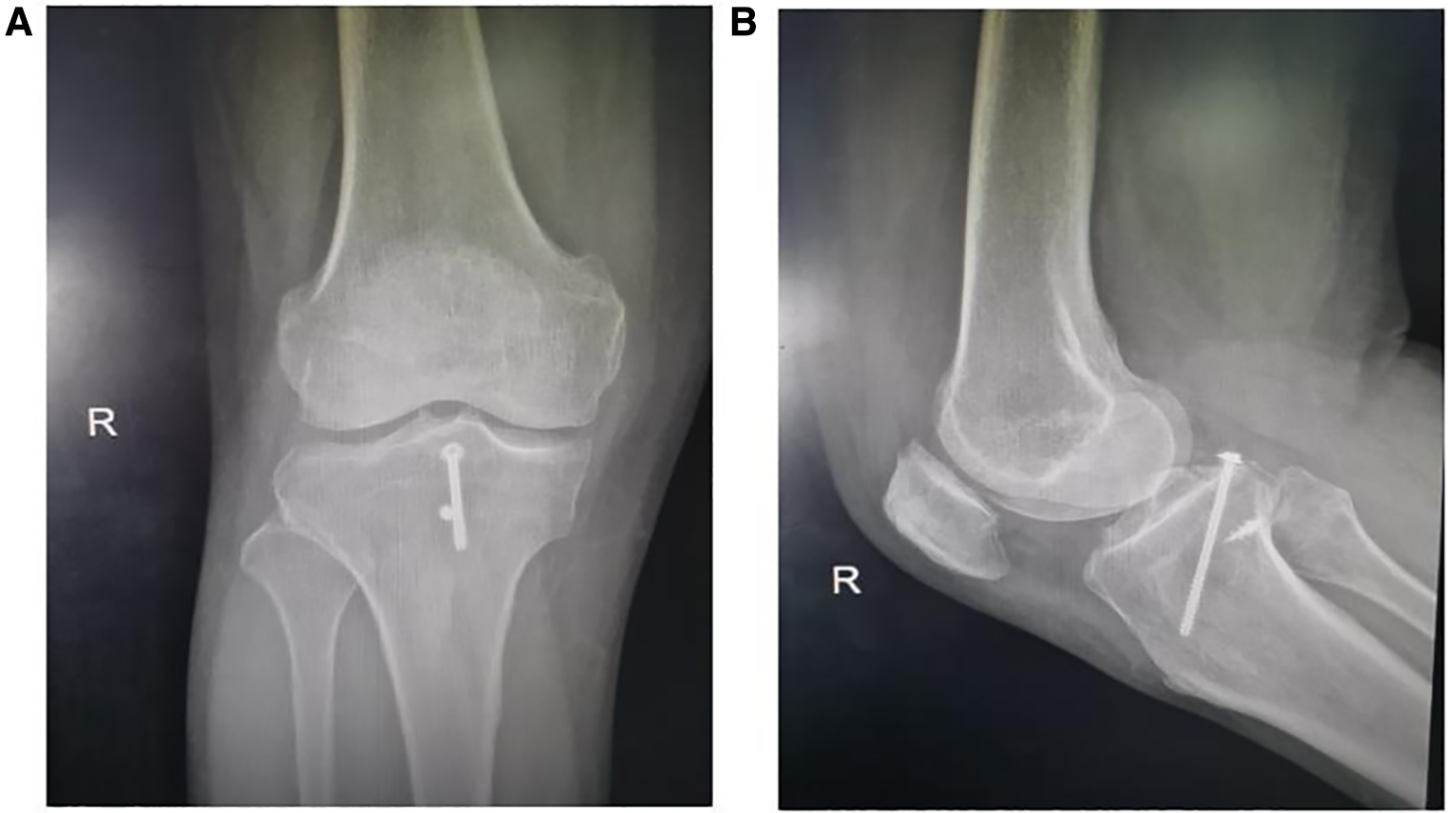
Postoperative radiograph of cannulated screw fixation (20 months). Follow-up x-rays showed that the avulsion fracture fragments of the tibial attachment point of the PCL healed well.
